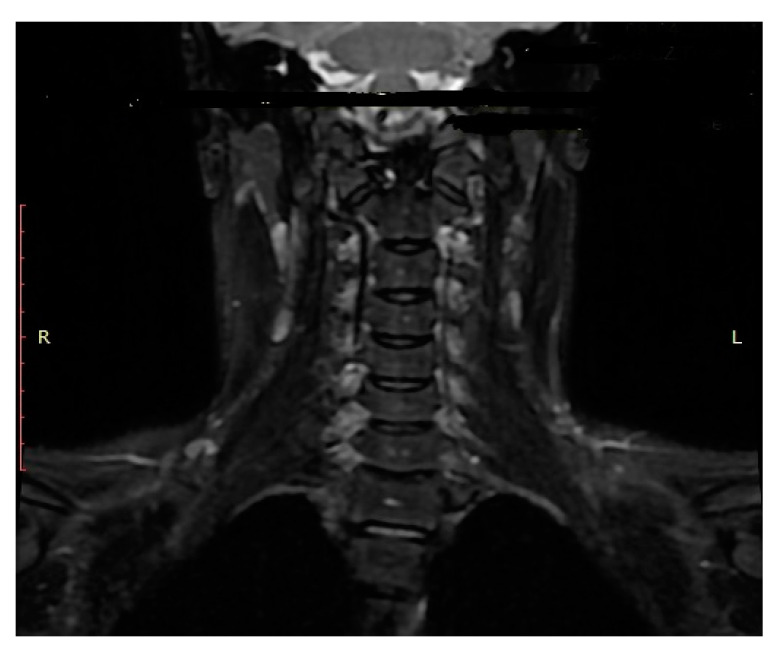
MRI scan (frontal plane) of a boy aged 15.2 years (patient: P.P.) confirming union of right-sided OCF in correct alignment.
